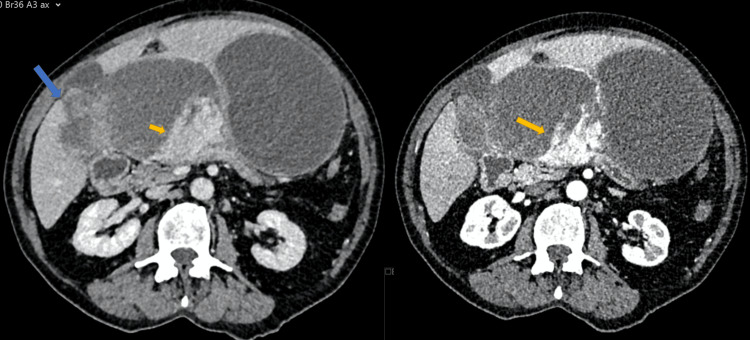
HA mimicking complex liver cyst Axial and coronal imaging of CECT showing the cystic lesion increased in size. On the right, a new solid tissue component infiltrating the liver (blue arrow) is evident along with a further increase in the inter-cystic soft tissue part of the lesion (yellow arrow). On the left, the inter-cystic soft tissue component is enhanced on arterial phase imaging (yellow arrow). HA: hepatic angiosarcoma; CECT: contrast enhanced computed tomography
